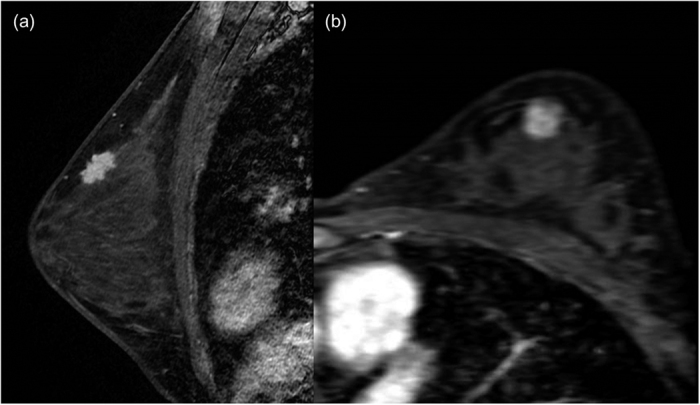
An infiltrating ductal carcinoma with focal ductal carcinoma *in situ* in the left breast of a 38-year-old woman. (a) Sagittal contrast-enhanced, non-subtracted, T1-weighted MR image with fat suppression shows a 2 cm spiculated mass. Note the tumor location in the fat-gland interface. (b) Subtracted axial reformatted image.
